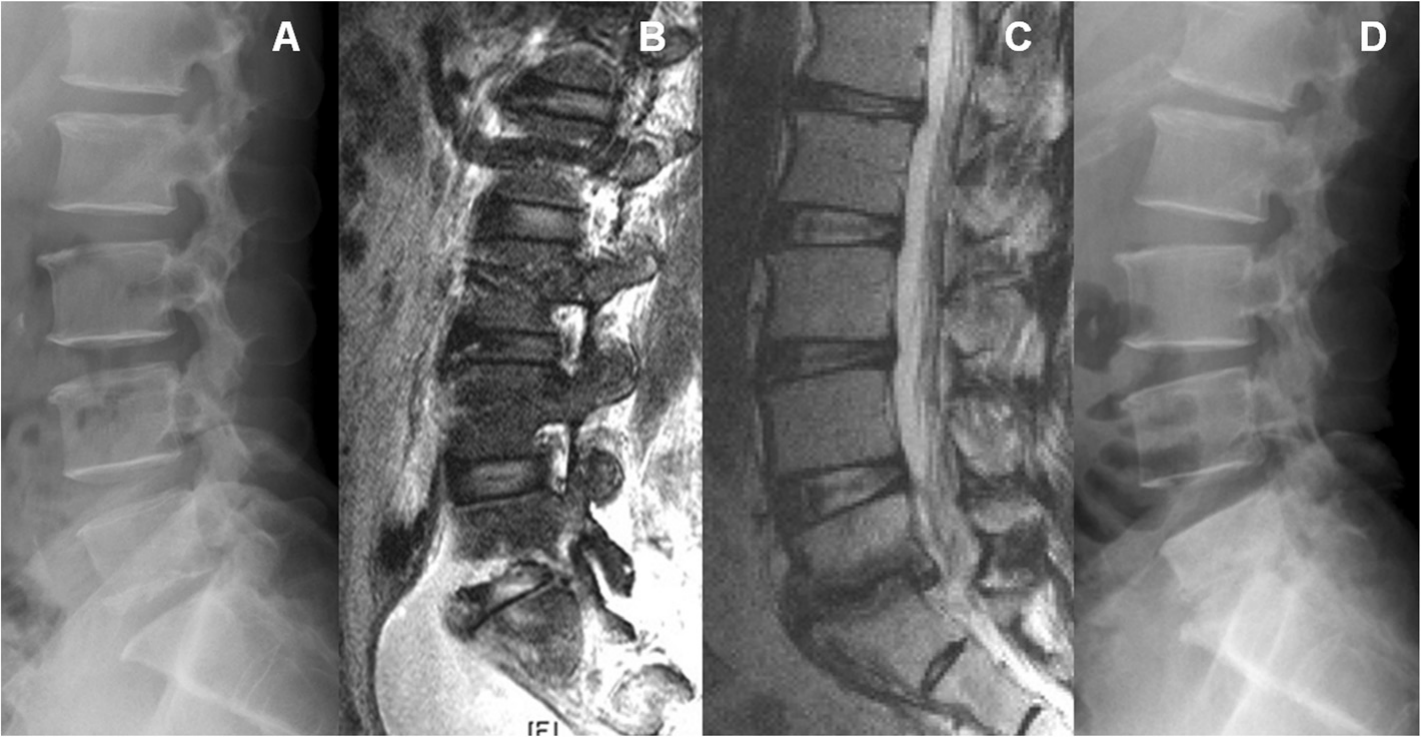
Presacral abscess treated by PEDI. L5-S1 infectious spondylitis was diagnosed in a male patient. The lateral radiograph showed L5 inferior endplate erosion (A). Sagittal T2-weighted MRI demonstrated presacral abscess (B). Postoperative sagittal T2-weighted MRI revealed the abscess was eradicated by PEDI (C). Postoperative lateral radiograph showed L5-S1 disc space collapse leading to spontaneous fusion (D).
